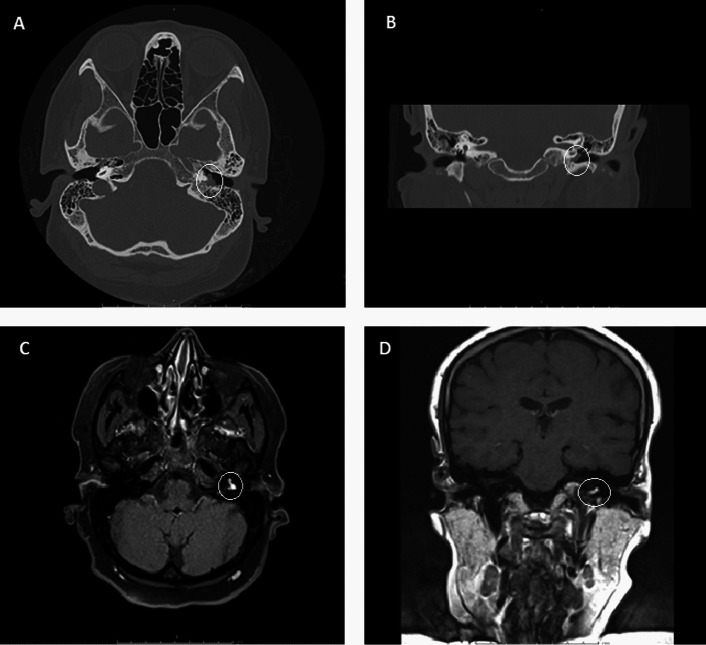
radiological evaluation of glomus tympanicum showing a left sided enhancing well-defined soft tissue mass (encircled) centered on the cochlear promontory. A HRCT axial view, B HRCT coronal view, C Gadolinium enhanced MRI axial view, D Gadolinium enhanced MRI coronal view
